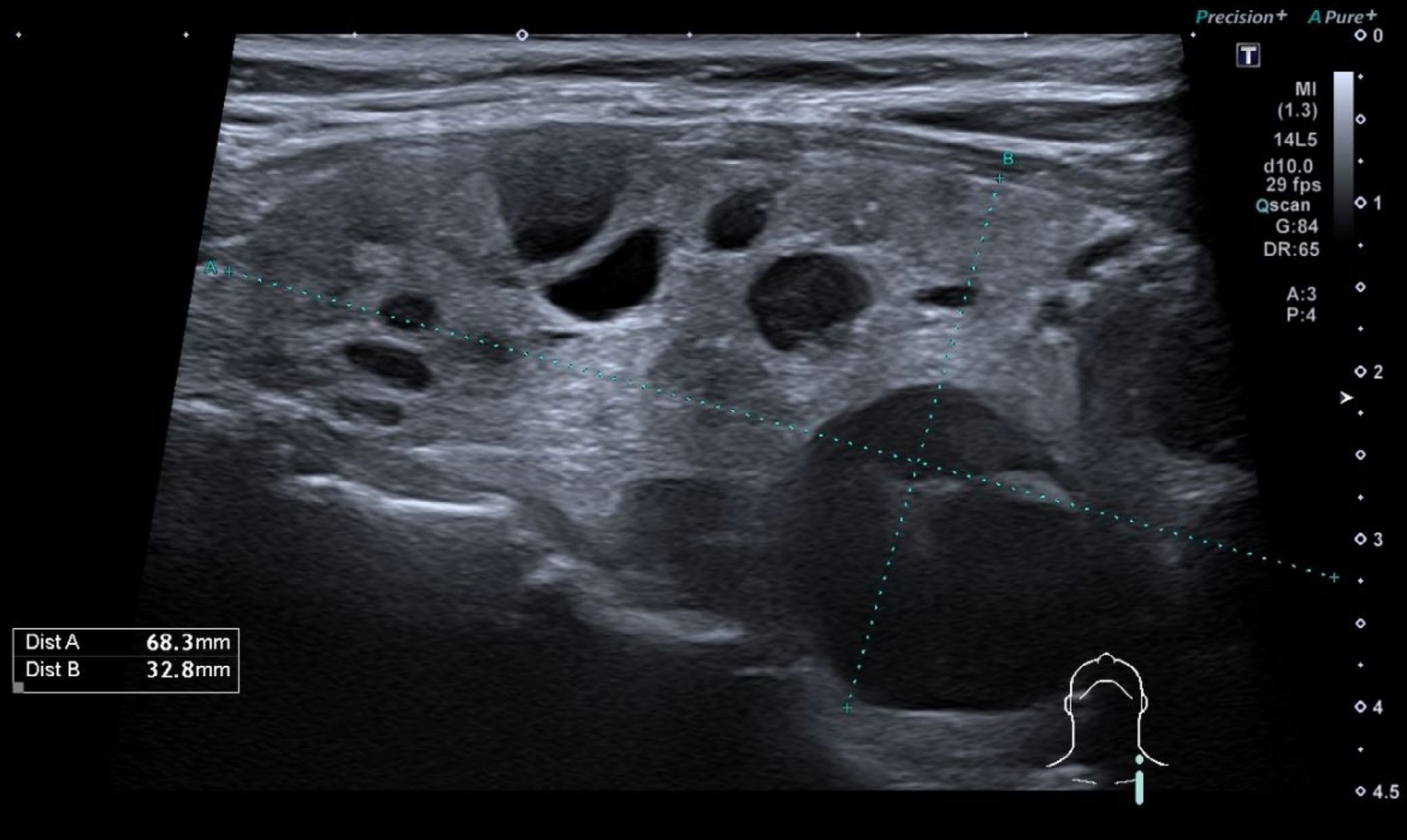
Thyroid ultrasound (longitudinal view); enlarged thyroid gland due to multiple cysts (largest of 23 mm).
